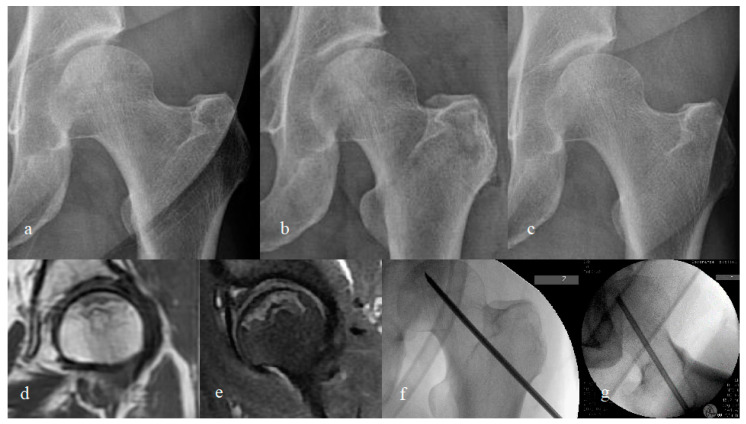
Images of the left hip of a woman who underwent multiple drilling and injections of recombinant human bone morphogenetic protein-2 (rhBMP-2) and β-tricalcium phosphate (β-TCP). (a) Pre-operative radiograph (hip anteroposterior view), taken on 3 April 2018; (b) immediate post-operative radiograph; (c) 3.2 year follow-up radiograph; (d) coronal T1-weighted magnetic resonance image; (e) sagittal T2-weighted magnetic resonance image; (f) intra-operative fluoroscopic image during multiple drilling; (g) intra-operative fluoroscopic image of small trephine used for the injection of rhBMP-2 and β-TCP.
